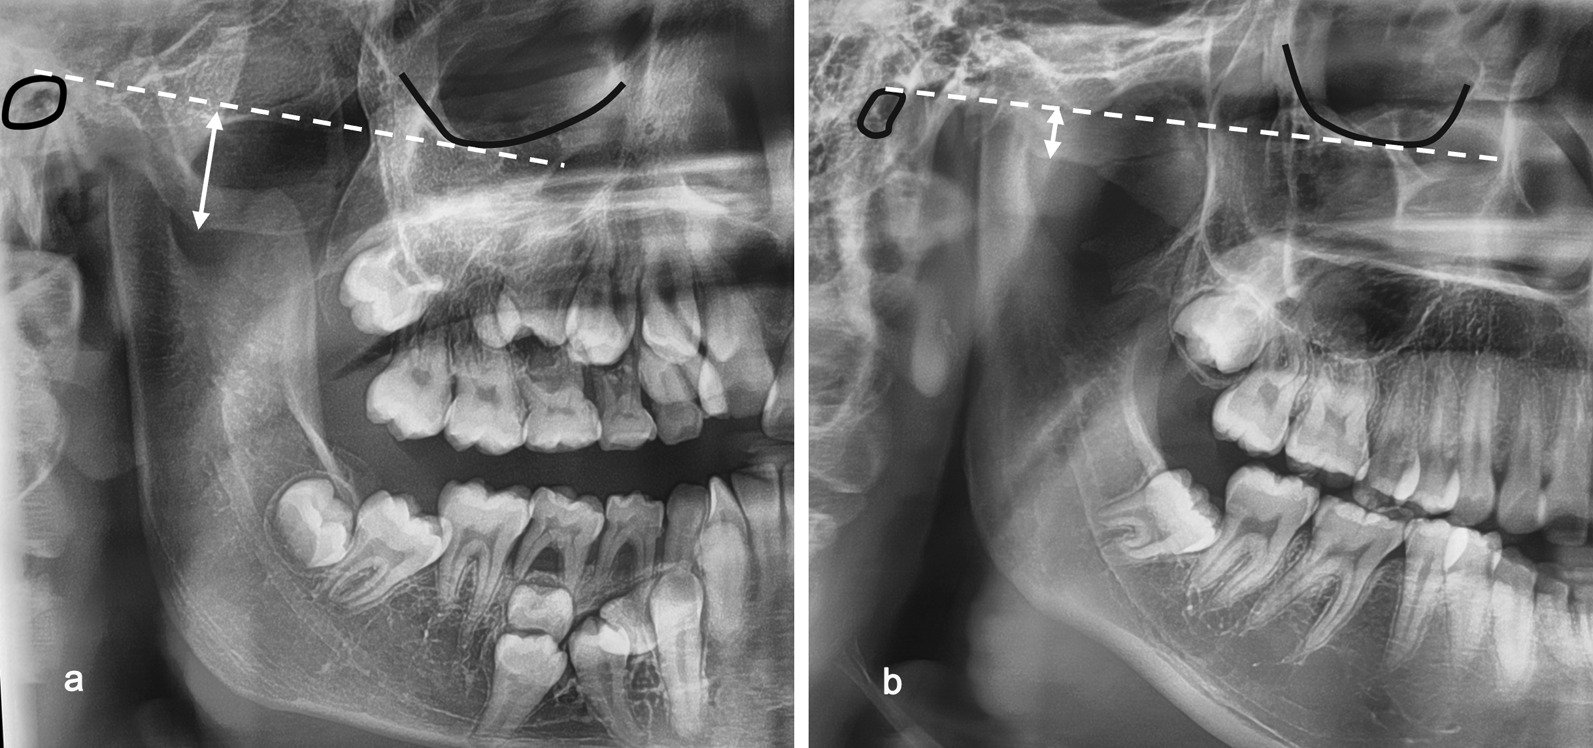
The ZAD observed in the panoramic radiograph. a ZAD in a CCD patient b normal ZAD in a matched control. The dotted line drawn from the porion to the orbitale indicates the Frankfort line
